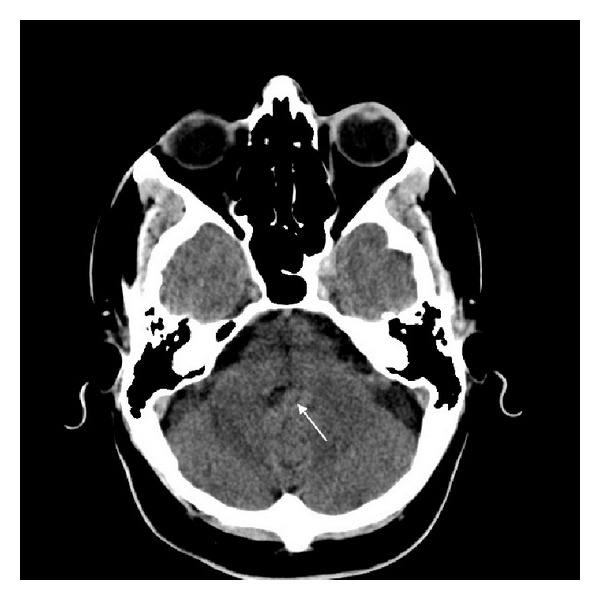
Brain CT scan demonstrating hyperdense tumor (arrow) filling the 4th ventricle.
